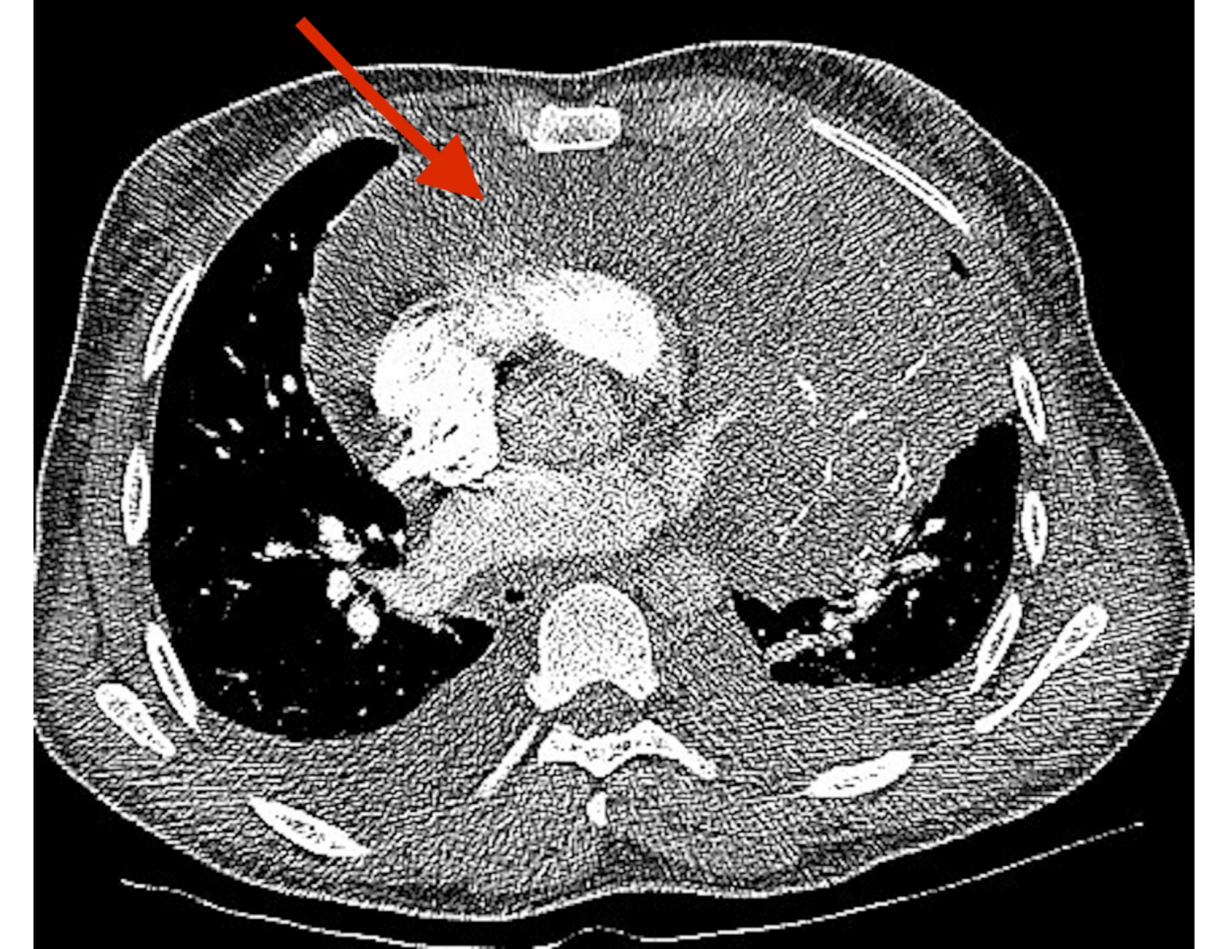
Computed tomography (CT) of the chest showing anterior mediastinal mass (arrow)
